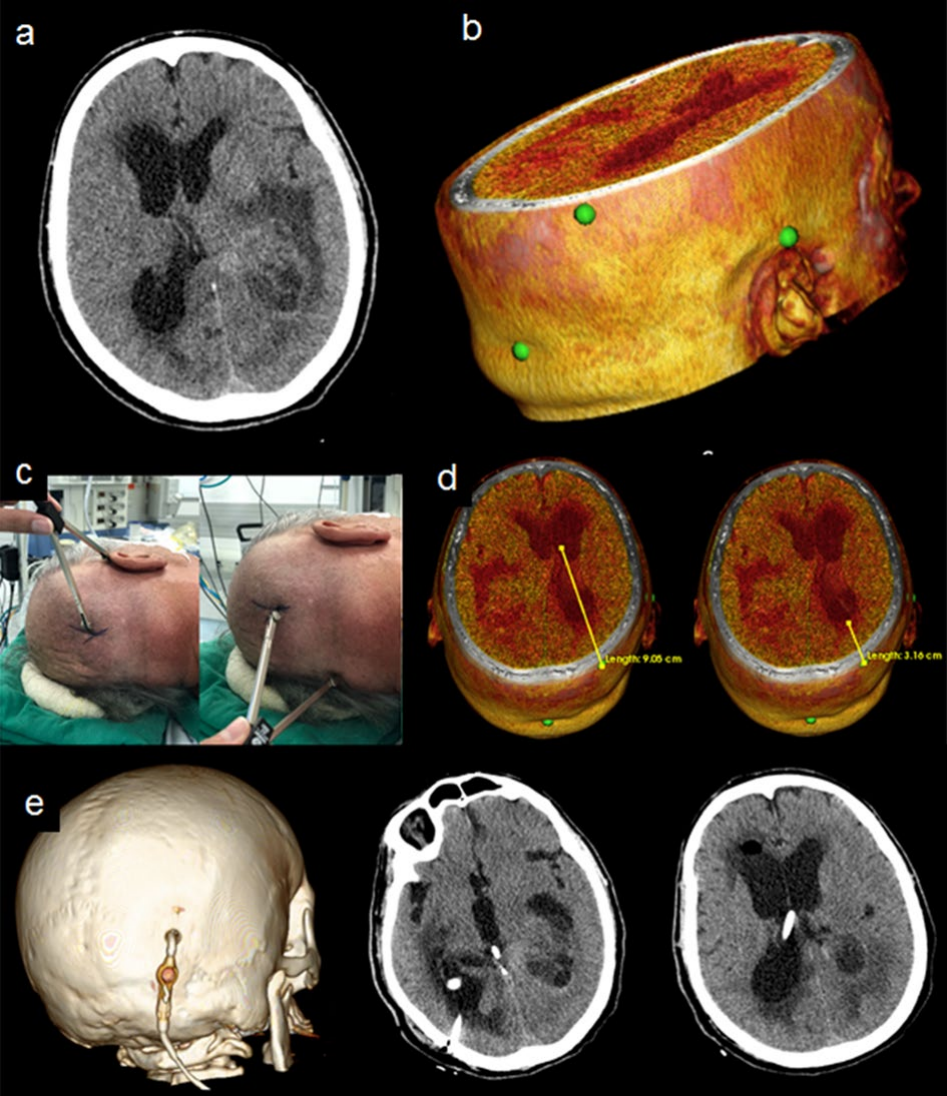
A 76-year-old patient diagnosed with an intraventricular tumor and hydrocephalus underwent VP shunt placement. (a) Cranial CT scan with a left intraventricular tumor and hydrocephalus; (b) 3DVR with the selected reference points and the target represented by green points; (c) image sequence showing head positioning and target identification using the compass (the target is represented by the intersection between the two lines drawn with the compass); (d) Surgical planning: distance between the target and the anterior horn of the right lateral ventricle and between the target and ventricular interior; (e) postoperative result.
